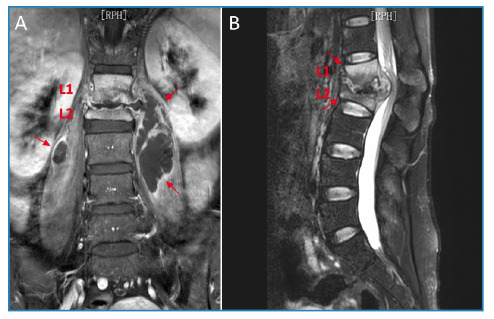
A: Magnetic resonance image (coronal section) showing apparent destruction of the L1 and L2 vertebrae accompanied by paravertebral abscesses (red arrows). B: Magnetic resonance image (sagittal section) showing obvious kyphosis resulting from significant destruction of the contiguous vertebral segments (L1 and L2 vertebrae) (red arrows).
